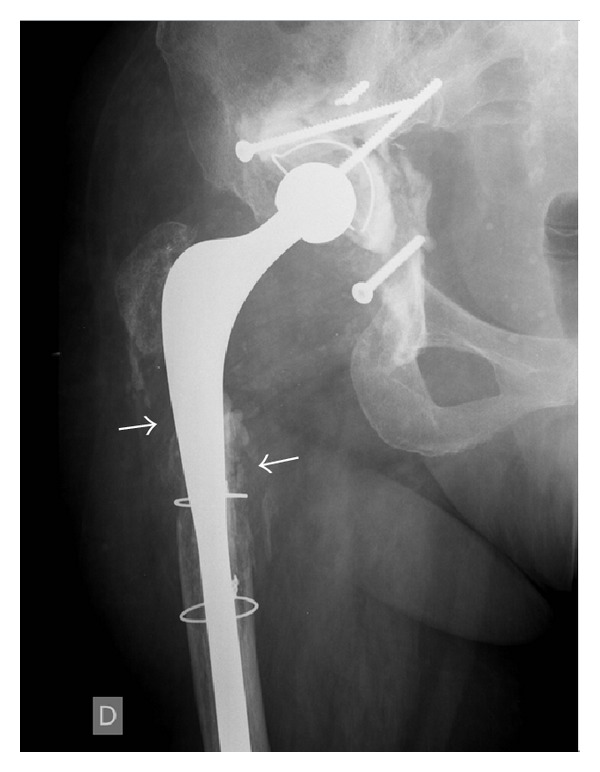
X-ray of total hip arthroplasty showing extensive lytic lesions around the femoral component (arrows), indicating infection.
